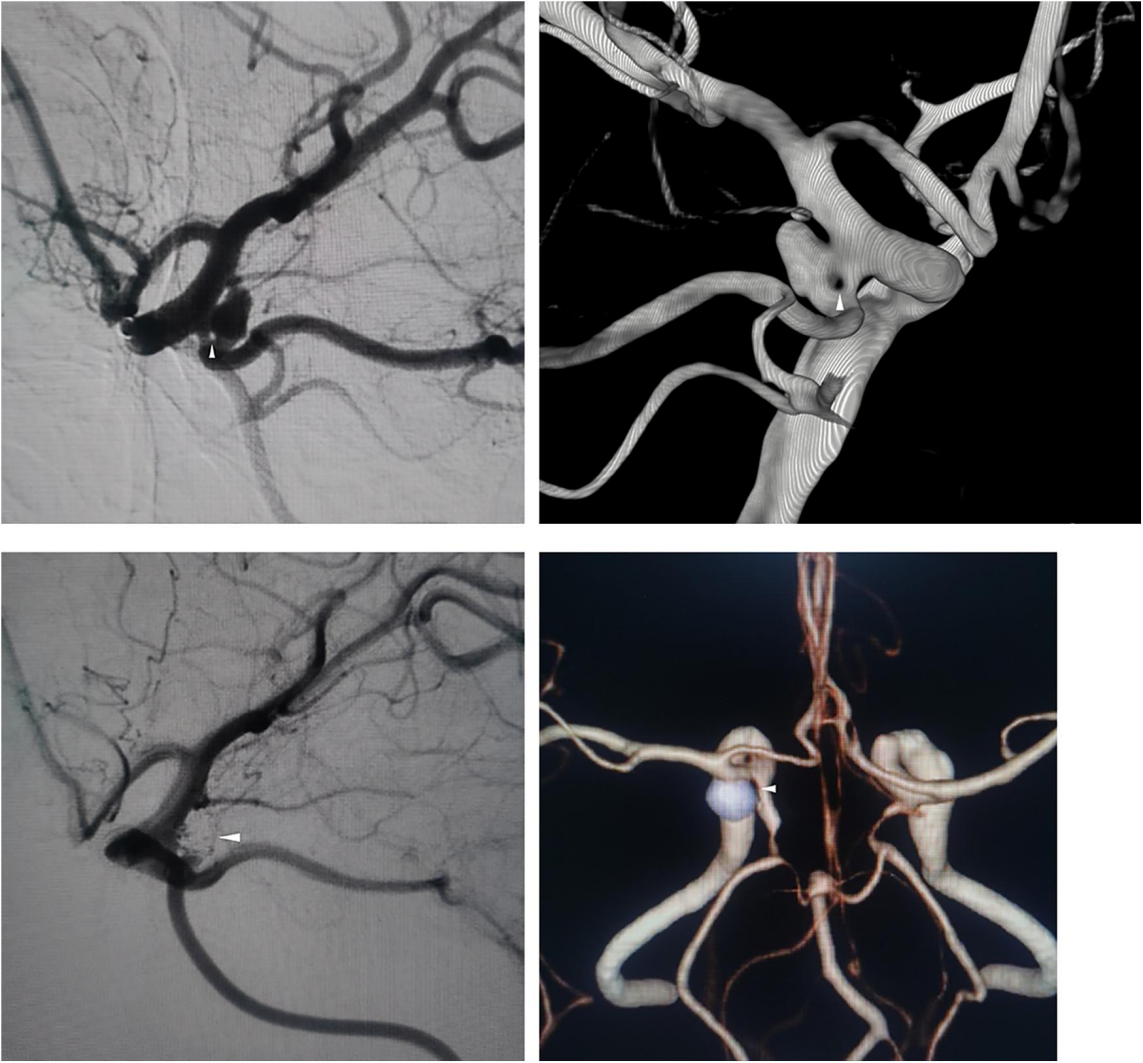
(A) Preoperative DSA shows left PCoA fenestration (arrow) and aneurysm. (B) 3D-RA confirms single-segment fenestration. (C) Postoperative DSA demonstrates complete aneurysm occlusion. (D) 12-month follow-up CTA confirms no recurrence.
